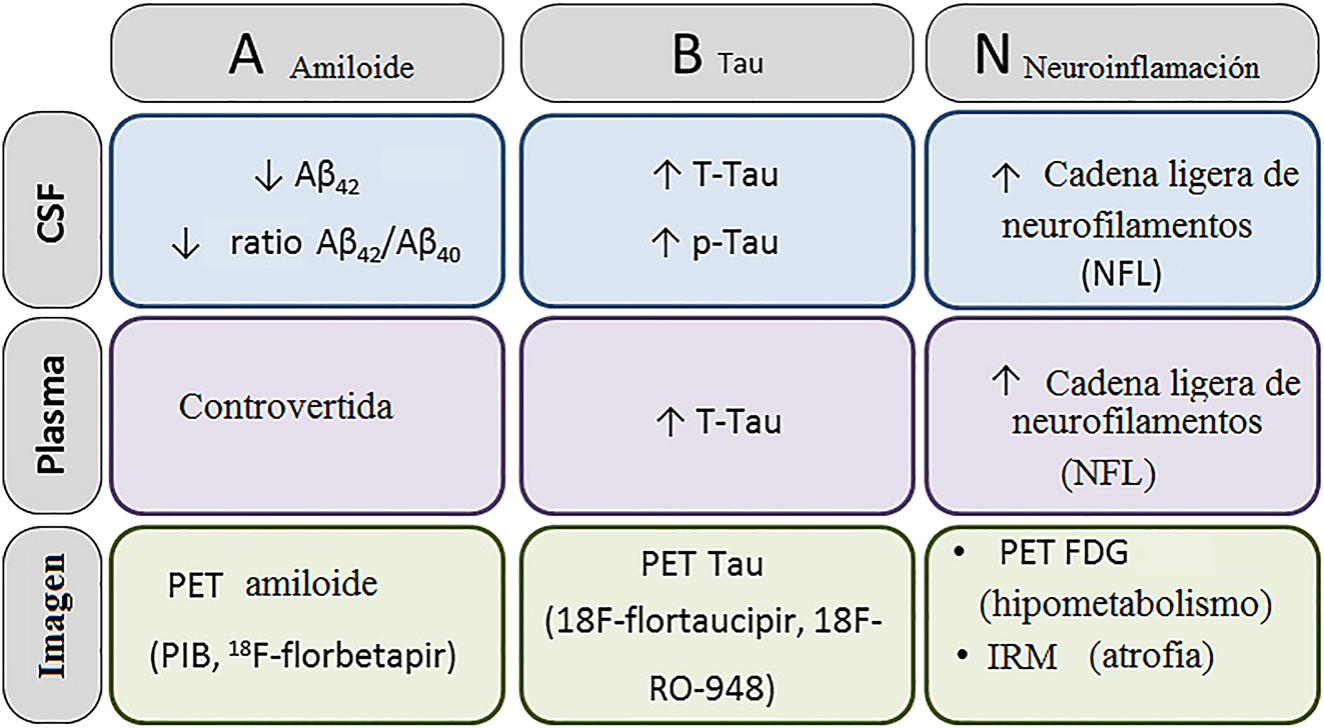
La clasificación de neurodegeneración basada en la proteína tau y el amiloide (AT(N)) define como biomarcadores “A” a la tomografía por emisión de positrones amiloide (PET), y a los niveles de Aβ42 y Aβ 42/40 en el líquido cefalorraquídeo (LCR). Los biomarcadores “T” son la proteína tau en PET y p-Tau en LCR, mientras que los biomarcadores “N” son la resonancia magnética estructural, el PET con fluorodesoxiglucosa (FDG), la tau total en LCR (t-Tau) y la proteína de cadena ligera de neurofilamentos (NFL).
